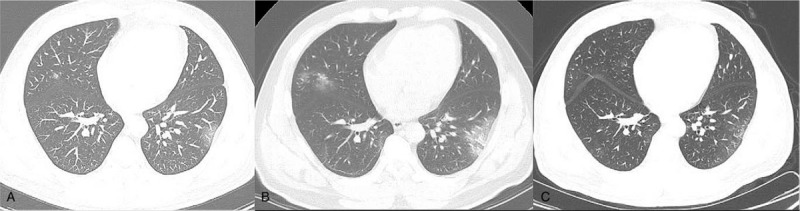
In a 46-year-old male with coronavirus disease 2019 by imported infection, the findings on initial computed tomography (CT) (day 1 after admission) are multiple subpleural focal ground glass opacities (GGOs) in both lungs (A). On day 8, the lesions manifest as larger-scale GGOs with great consolidation in some previous GGOs in both lungs on the follow-up CT images (B). On day 12, the ranges of lesions have reduced in a great extent, and CT images show strip-like opacity (C).
